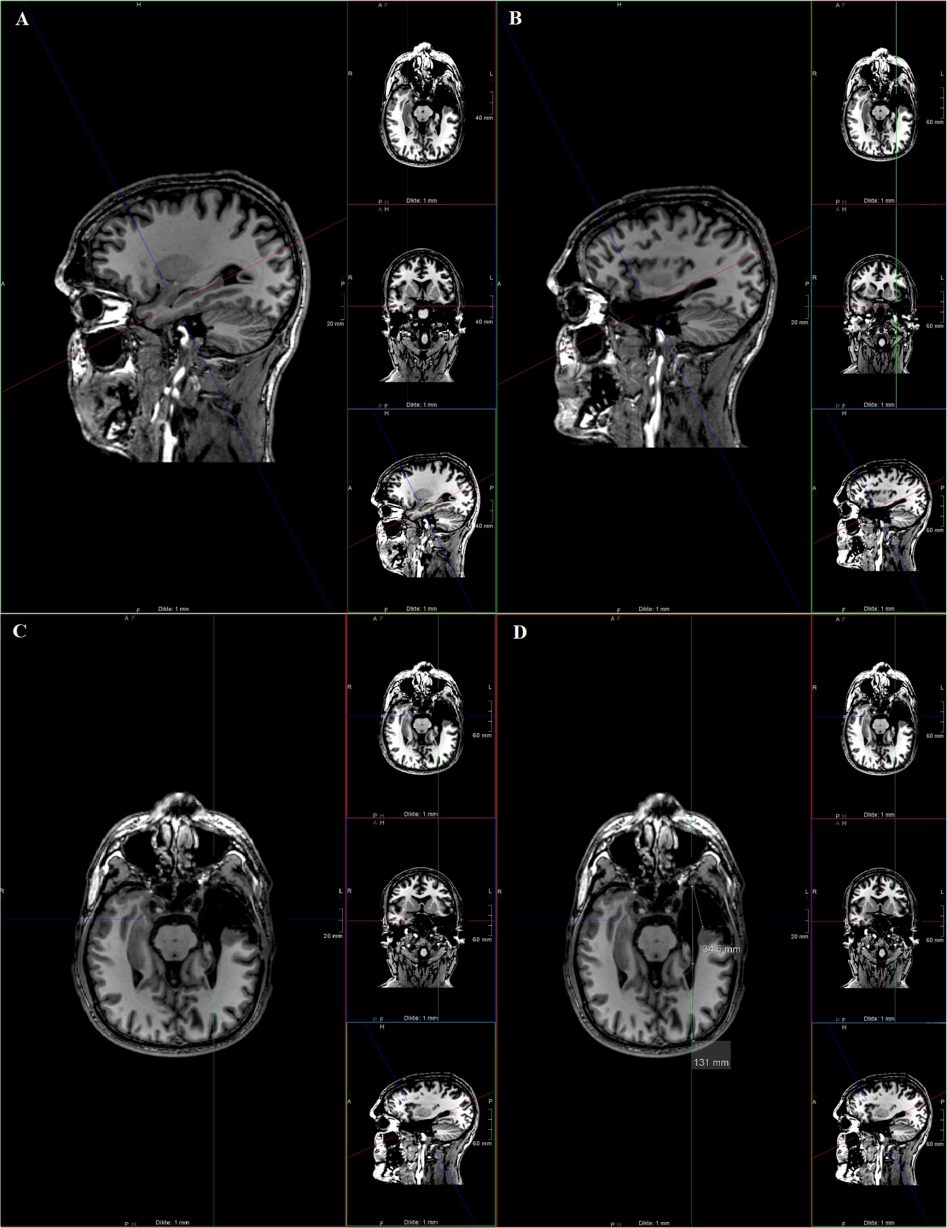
To measure the extent of resection, we reconstructed the 3D T1 scan in a plane parallel to the hippocampus at the non-resected side, as shown in a. Next, we returned to the resected side within the same plane (b) and switched back to the axial view (c). Finally, the anterior-posterior (AP) length of resection was estimated by measuring the distance from the anterior tip of the middle sphenoid fossa (which had contained the resected temporal pole) to the posterior margin of the resection cavity in axial view. To compensate for variations in head size and possible metrical distortion introduced by MRI, the extent of resection was expressed as a fraction of the distance between the anterior tip of the middle sphenoid fossa to the occipital pole (anterior temporal-occipital pole, or ATOP distance), shown in d
